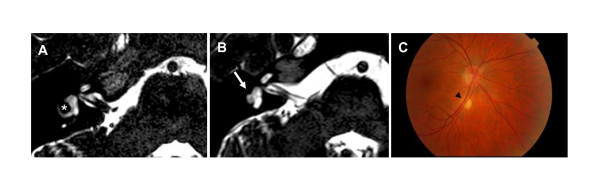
The axial 3D T2-weighted MRI images from the region of the inner ear of (A) a KS patient with normal semicircular canals (asterisk), and (B) a KS patient with hypoplastic semicircular canals (arrow), and an unspecified atrophic area (arrow head) in his retina (C).
